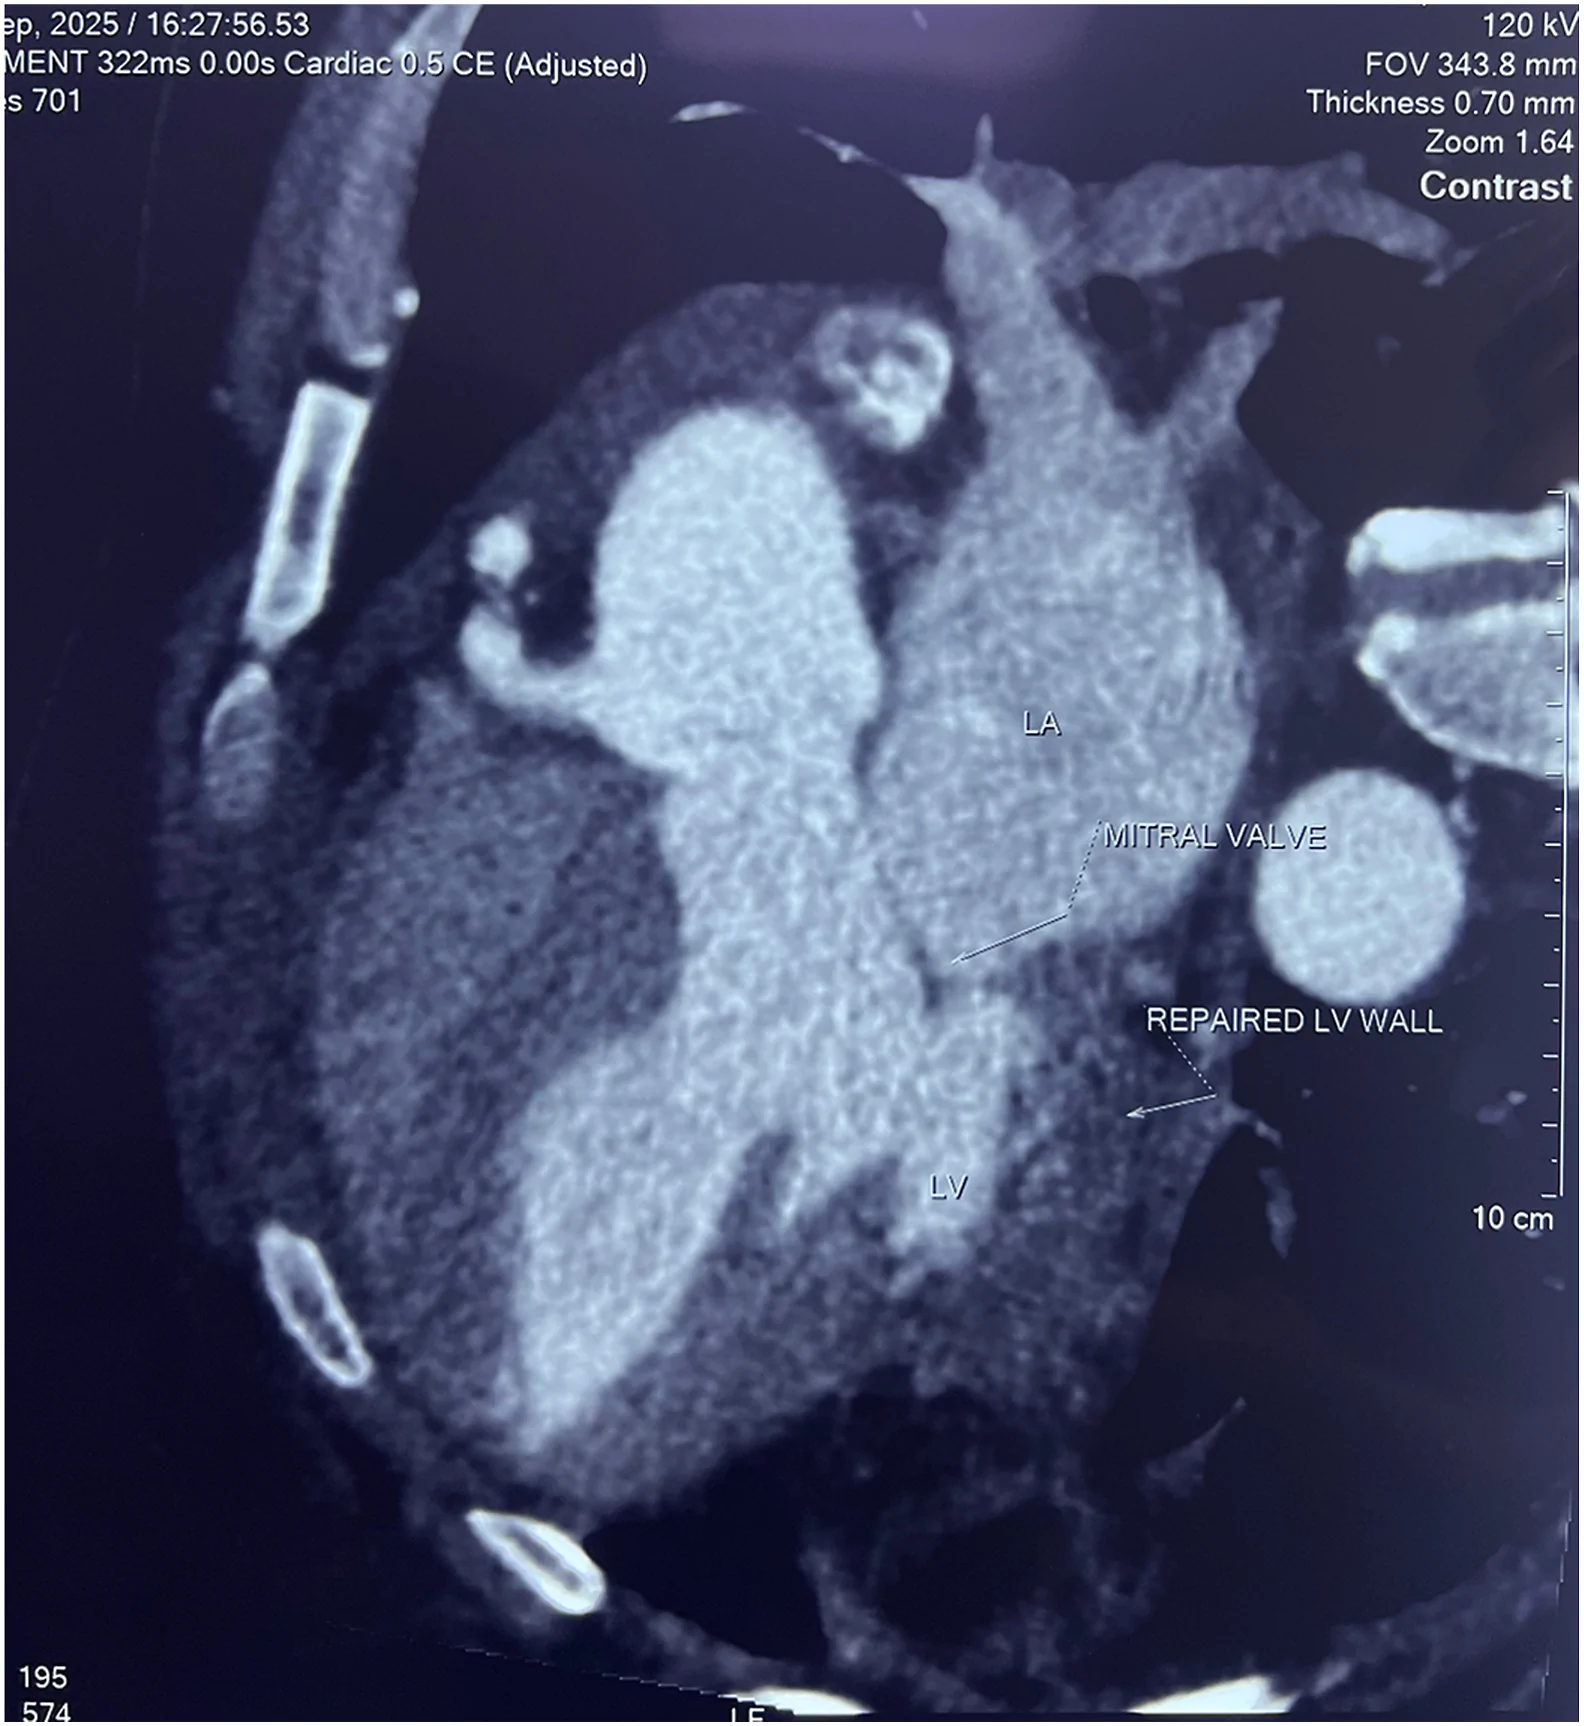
Cardiac computed tomographic angiography showing the repaired mitral valve and restored left ventricular (LV) geometry. (FOV, field of view; LA, left atrium.)
